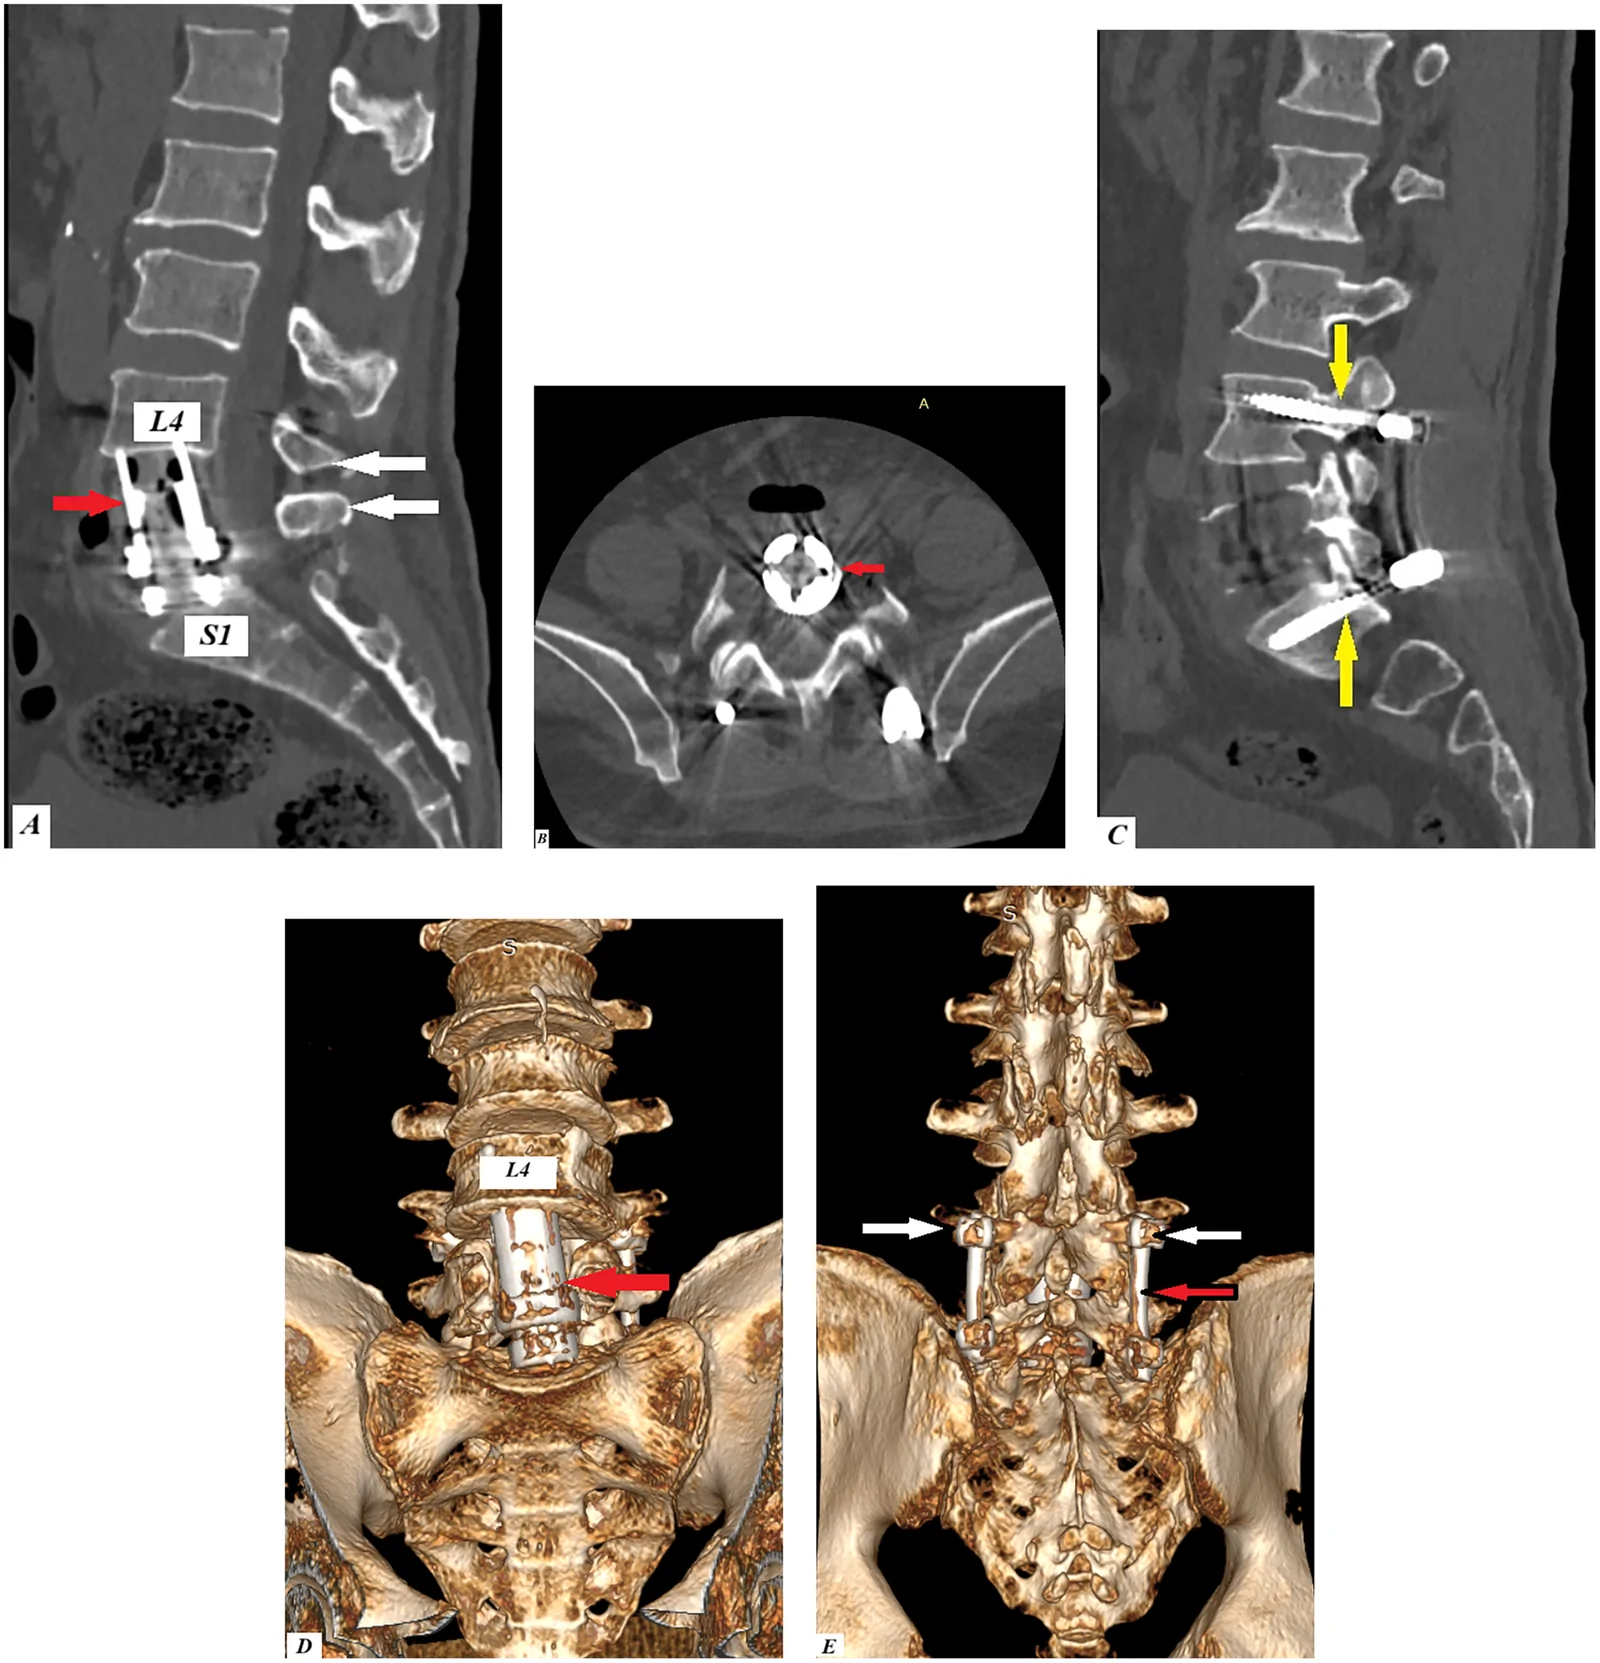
Postoperative lumbar spine CT images (A–C) and 3D reconstructions (D,E). (A) Sagittal plane. The red arrow indicates the VLIFT titanium cage placed instead of the resected L5 vertebra. The white arrows point to the spinous processes of L4 and L5, confirming preservation of the posterior column. (B) Axial plane. The red arrow shows the centrally positioned VLIFT titanium cage. (C) Sagittal plane. The yellow arrows indicate cannulated screws inserted into the pedicles of L4 and S1. (D) Anterior 3D reconstruction. Total resection of the L5 vertebral body is visualized. The red arrow highlights the VLIFT titanium cage. (E) Posterior 3D reconstruction. The posterior column is completely preserved. The white arrows show the pedicle screws of L4, and the red arrow indicates the connecting rod.
